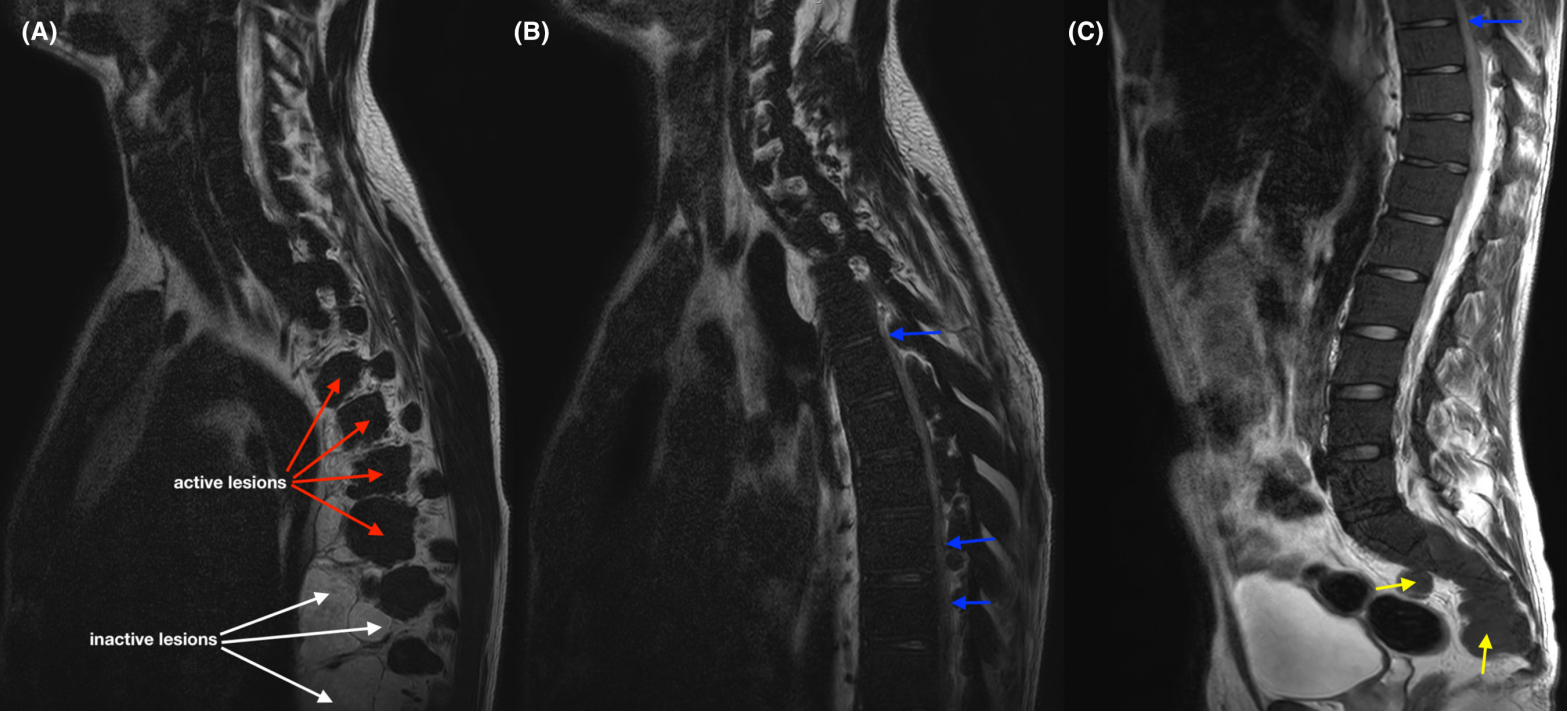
Sagittal T2‐weighted MRI: (A) thoracic paravertebral lesions—active lesions (red arrow) show intermediate signal intensity while older inactive lesions (white arrow) show high signal intensity due to fatty infiltration^1^; (B and C) narrowing of the spinal canal and compression of the medulla along the thoracic spine by lesions inside the spinal canal (small blue arrows); (C) lumbosacral active sites of extramedullary hematopoiesis (small yellow arrows).
